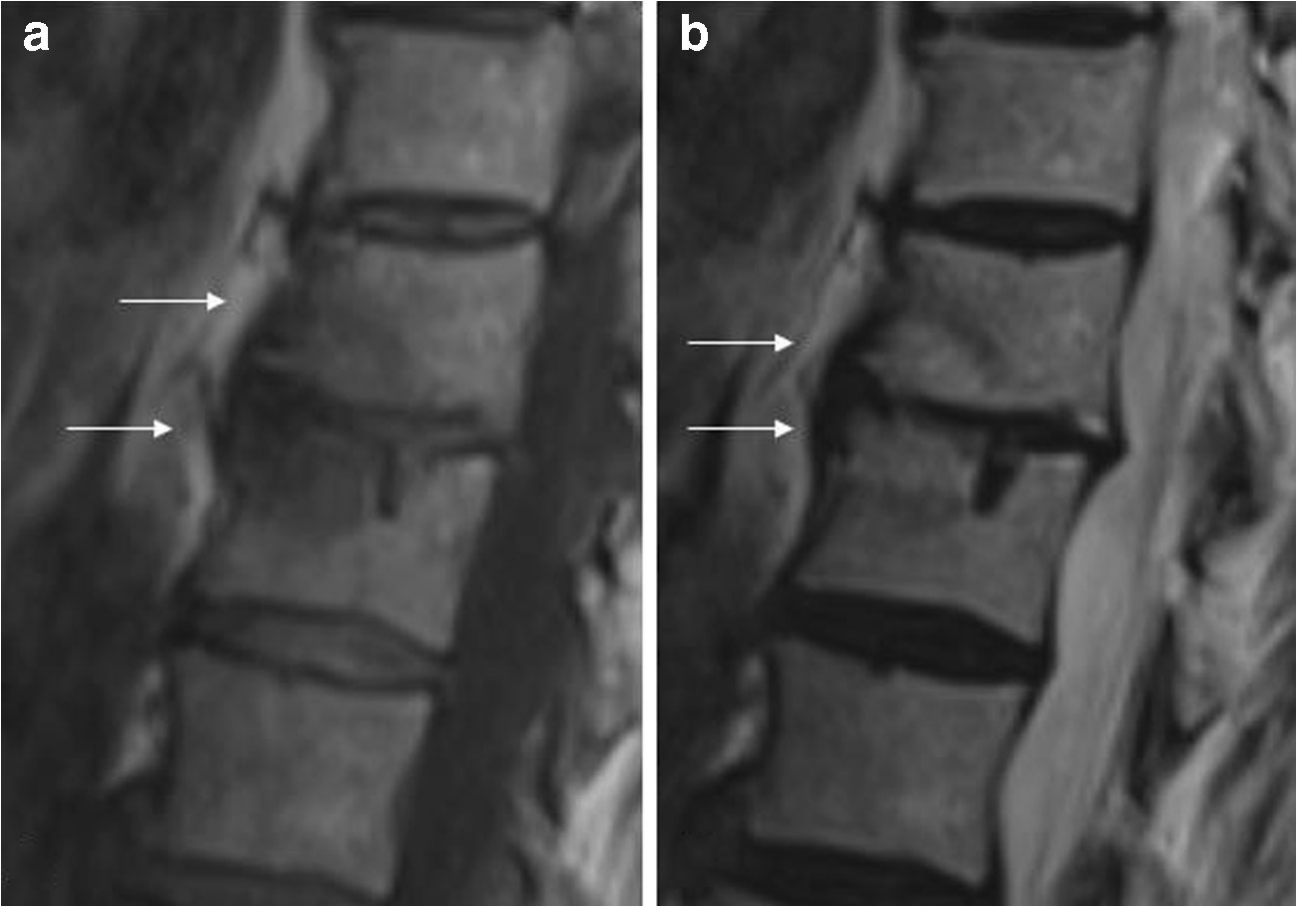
a, b Degenerative. Modic changes type I in a 78-year-old woman with back pain. MRI shows the SI alterations in D11 and D12 endplates (*arrows*), that appear hypointense on T1w (a) and hyperintense on T2w (b) imaging. These alterations correspond to vertebral body edema and hypervascularity
